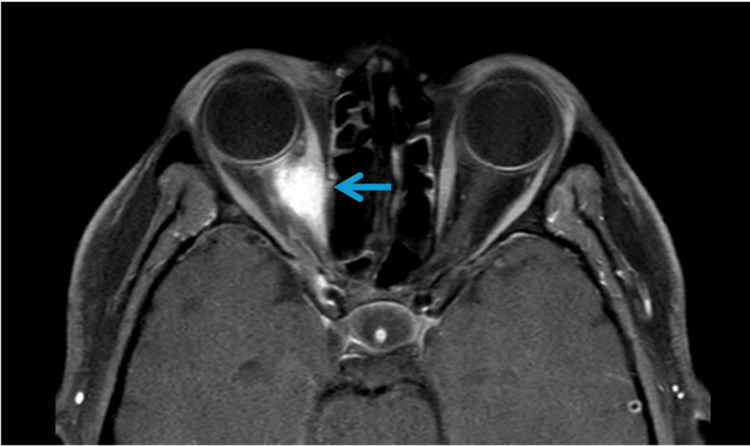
Right orbit metastases MRI orbits: within the right orbit, an enhancing mass involves the medial rectus and superior oblique and exerts mass effect on the optic nerve, resulting in deformation. Enhancement of the optic nerve suggests inflammation or infiltration.
